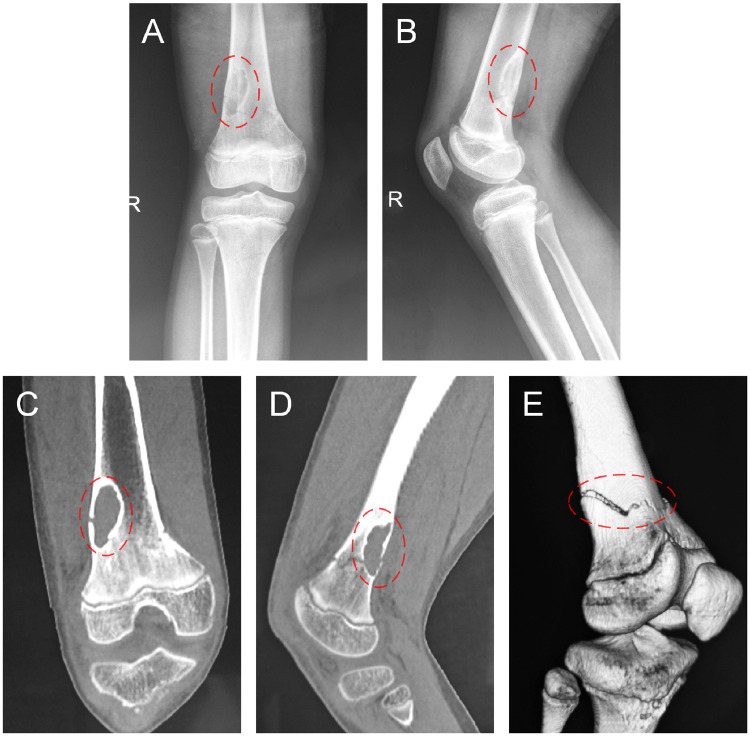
Preoperative imageological examinations of the patient. An oval shadow was located at distal of femur on frontal (A) and lateral (B) views of plain radiography; evident cavity was shown on coronal (C) and sagittal (D) CT images; an obvious pathological fracture could be observed on 3D reconstruction of CT (E). (the lesion is noted by dashed circle in each picture).
